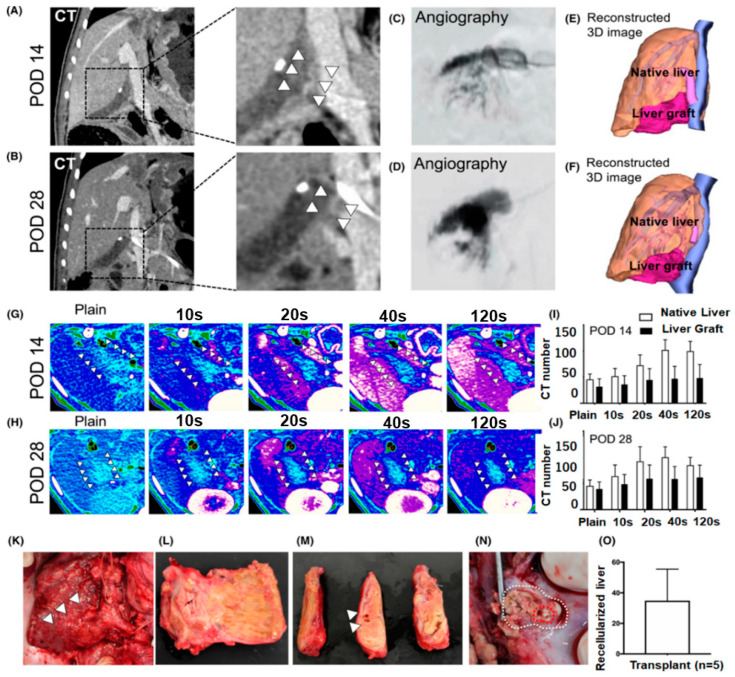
Engraftment (in vivo) of the bioengineered liver graft at different time points (postoperative days 14 and 28). (A,B) CT images of the transplanted graft on postoperative 14 (A) and postoperative 28 (B). Angiography through the intraportal infusion catheter on postoperative 14 (C) and postoperative 28 (D). Reconstructed three-dimensional CT image of the remnant native liver and the transplanted graft on postoperative 14 (E) and postoperative 28 (F). Time courses of the CT images for the transplanted graft on postoperative 14 (G) and postoperative 28 (H), Blue tube depicts IVC and HV, and pink tube depicts PV. Time course of the mean CT numbers for the graft and remnant native liver on postoperative 14 (I) and postoperative 28 (J). Intraoperative images of the graft on postoperative 28 (K,L). Macroscopic images of the procured graft on postoperative 28 (M). The arrowheads indicate PV in the graft. Macroscopic image of the stenosed anastomosis site of the procured graft on POD 28 (N). Patency rate was estimated to be approximately 11.9%. The dashed red and white lines show the anastomosis site and patent area, respectively. Weight of the procured graft on postoperative 28 (O). Figure 5 is adopted with copyright permission from [156], Elsevier.
